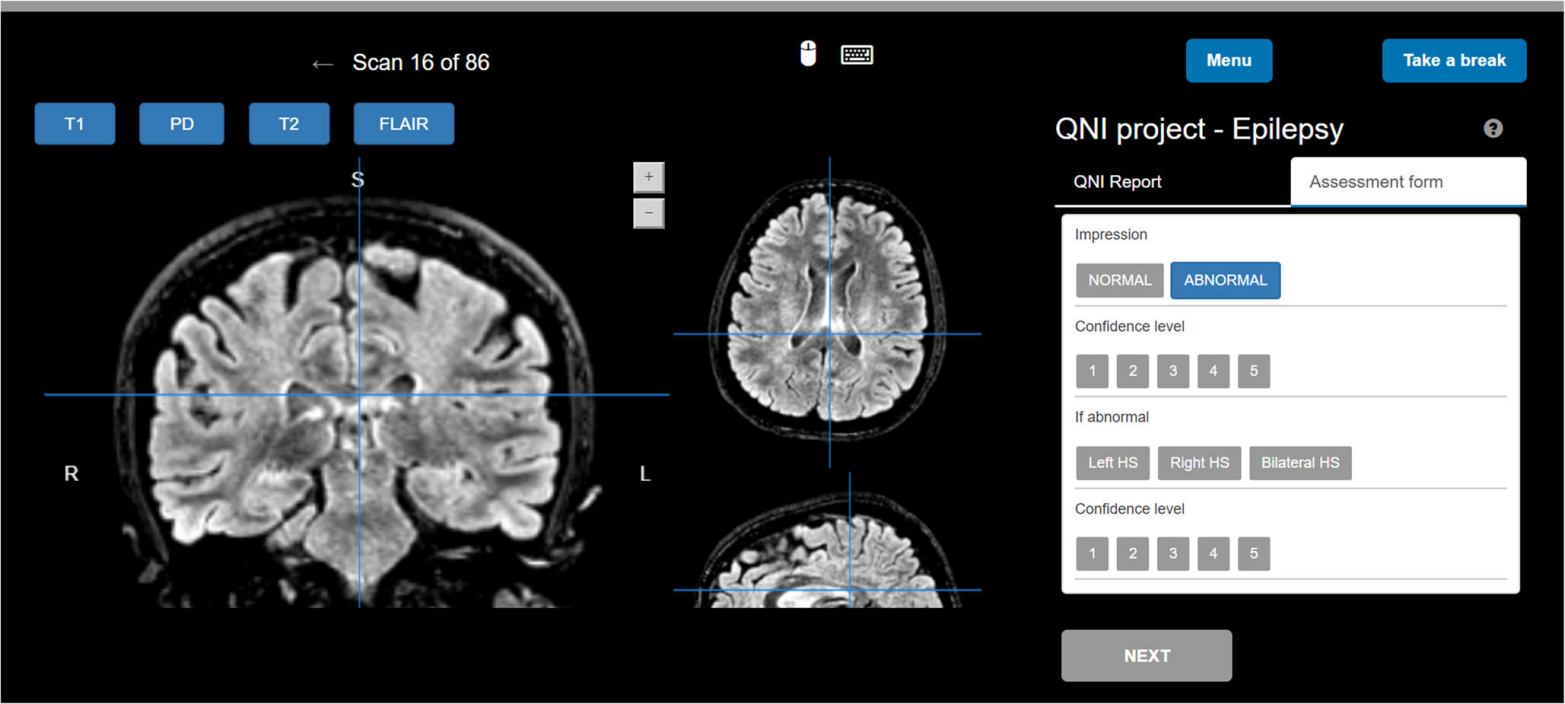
Snapshot of the website platform where raters performed their assessments. T1, PD, T2, and FLAIR sequences were available in interchangeable panels. The assessment form is seen on the right, which was either available by itself or tabbed alongside a QReport
